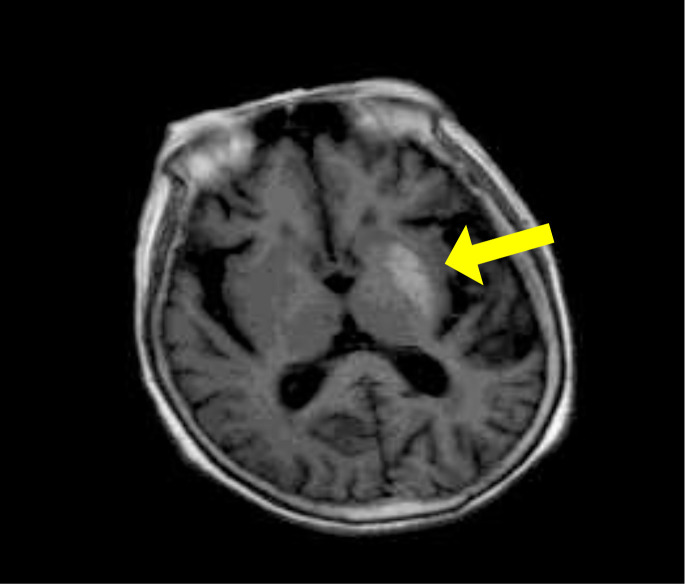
T1-weighted images (T1WI): Patchy slightly hyperintense signal primarily in the left basal ganglia (globus pallidus and putamen) (indicated by yellow arrow).
